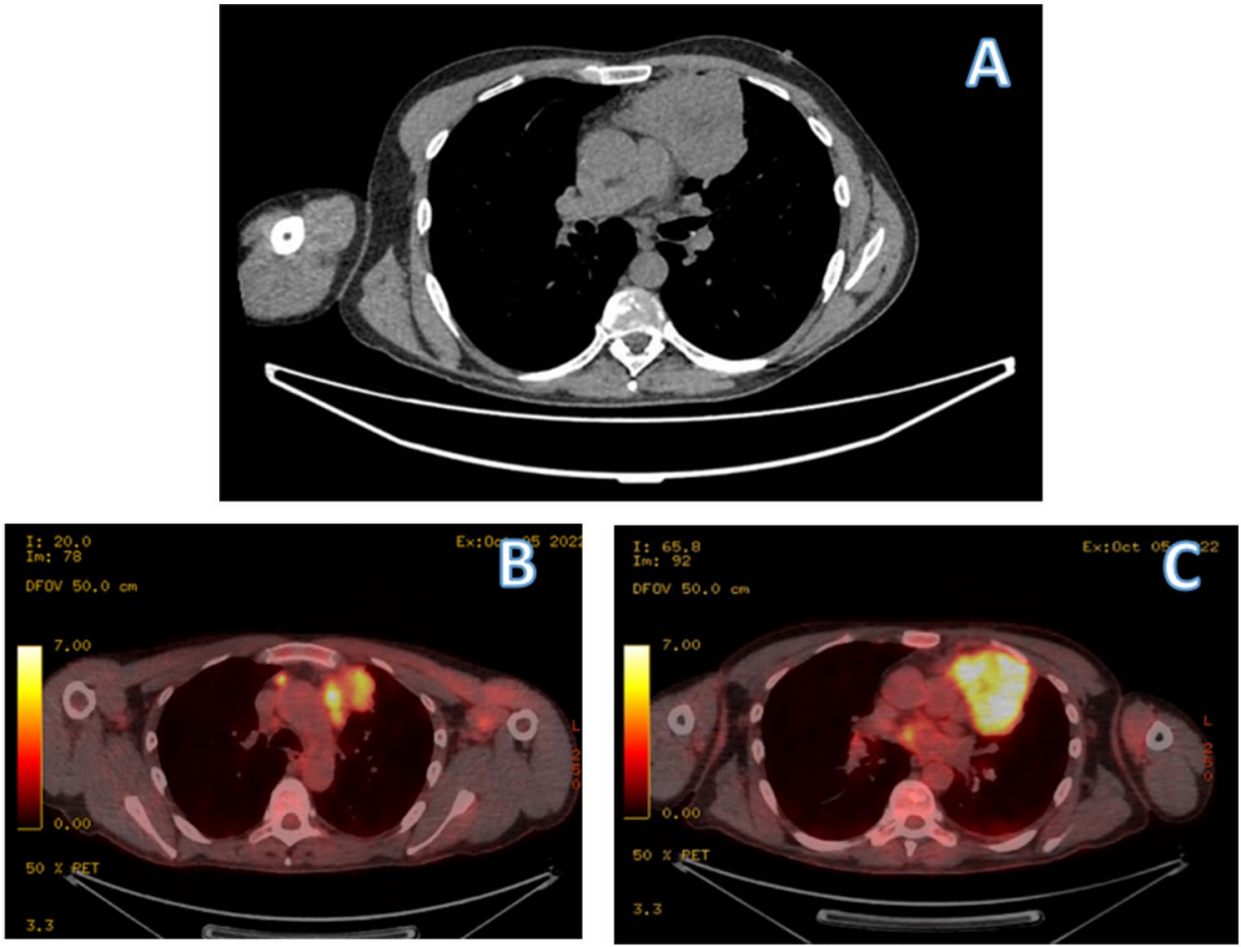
A Axial CT scan demonstrating left anterior mediastinal mass B & C Axial PET showing FDG avid right thymic nodule and left anterior mediastinal tumour involving the left upper lobe
